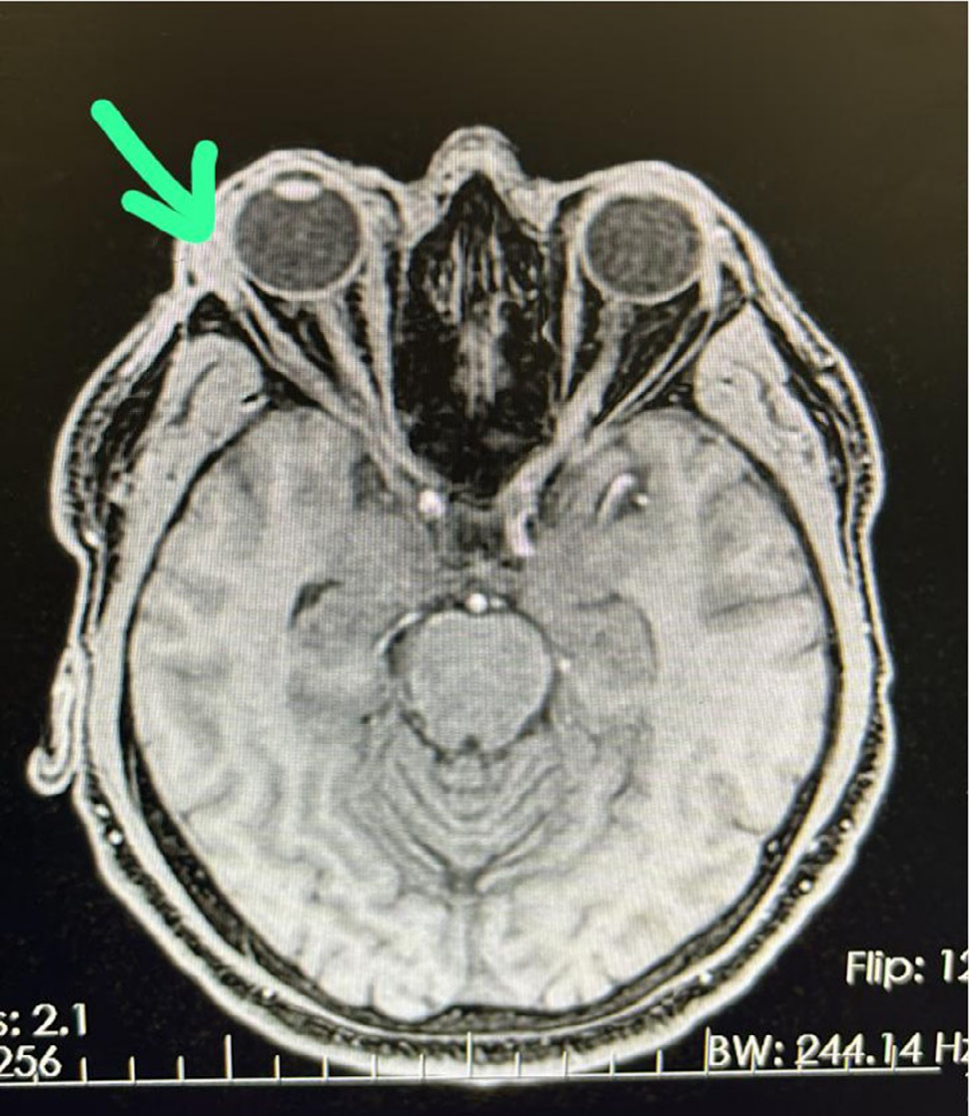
Orbital MRI showing bilateral edema and increased signal intensity of the ocular muscles, more prominent on the right side, with enhancement and thickening of the inflamed extraocular muscles (green arrow) and mild right orbital proptosis.
